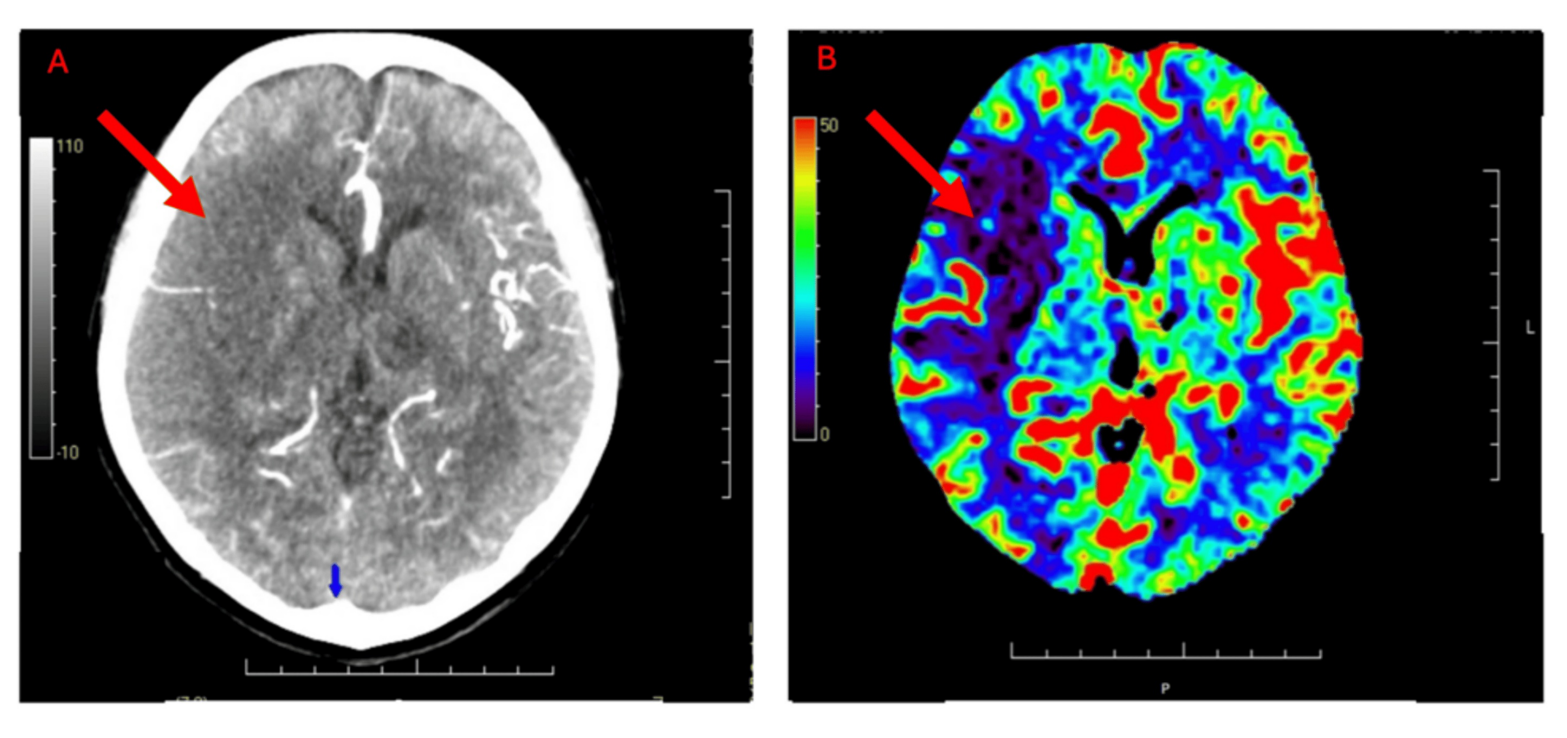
CT stroke study: cerebral CT and perfusion imaging. (A) A non-contrast CT scan of the head shows an area of cortical hypoattenuation and loss of gray–white differentiation in the right frontal region (red arrow), suspicious for acute ischemia. (B) CT perfusion map demonstrates reduced cerebral blood flow (red arrow) in the corresponding right frontal region, consistent with acute hypoperfusion.
